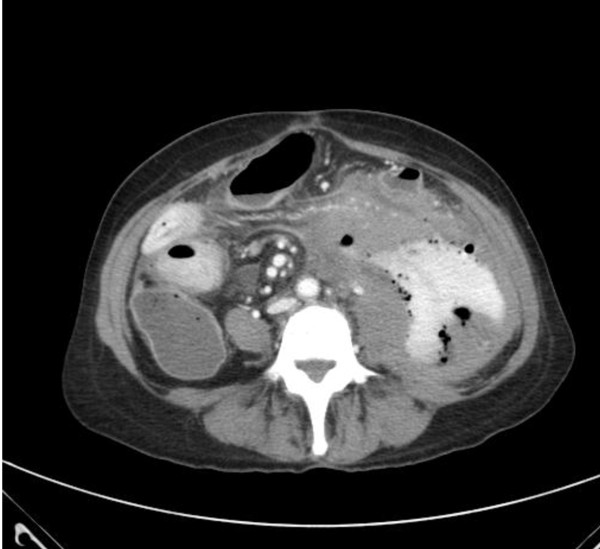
A computed tomography (CT) scan of the abdomen and pelvis showing loculated extravasation of contrast and air consistent with a ruptured viscus.
